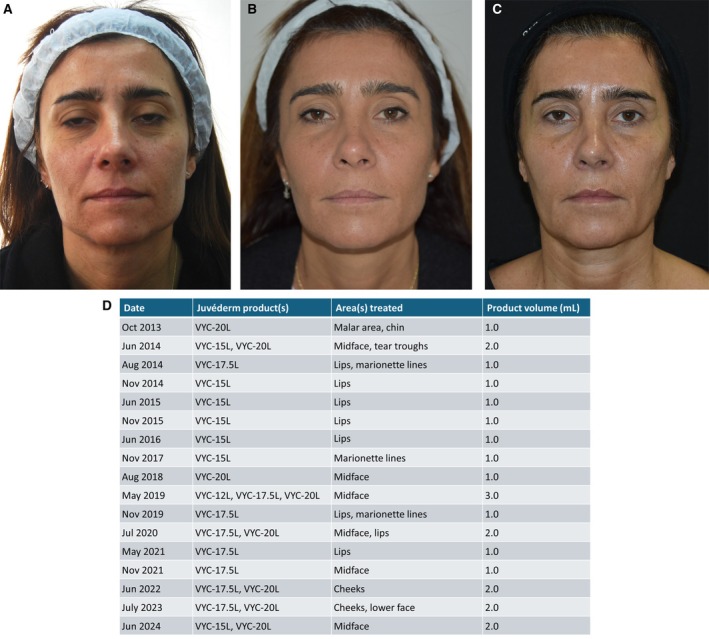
Long‐term natural results with Juvéderm used for 11 years. Part A shows a female patient aged 44 years, prior to treatment with Juvéderm products. Part B shows her 4 years later, and part C shows her 12 years later—aged 56 years—following multiple rounds of injections (1 year since the most recent). Her treatment plan is summarized in part D. Over this period, she also received long‐term neuromodulator injections (UFLs, DAO, platysma, chin) and intense‐pulsed light treatment, as well as occasional microfocused ultrasound. Patient images are courtesy of author G.C. DAO, depressor anguli oris; UFL, upper facial line.
